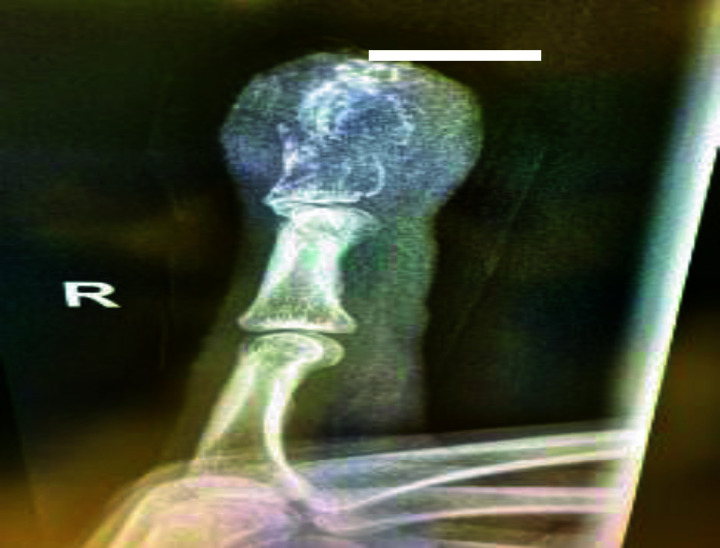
Plain X-ray of right index finger showing an ill-defined lytic lesion.
